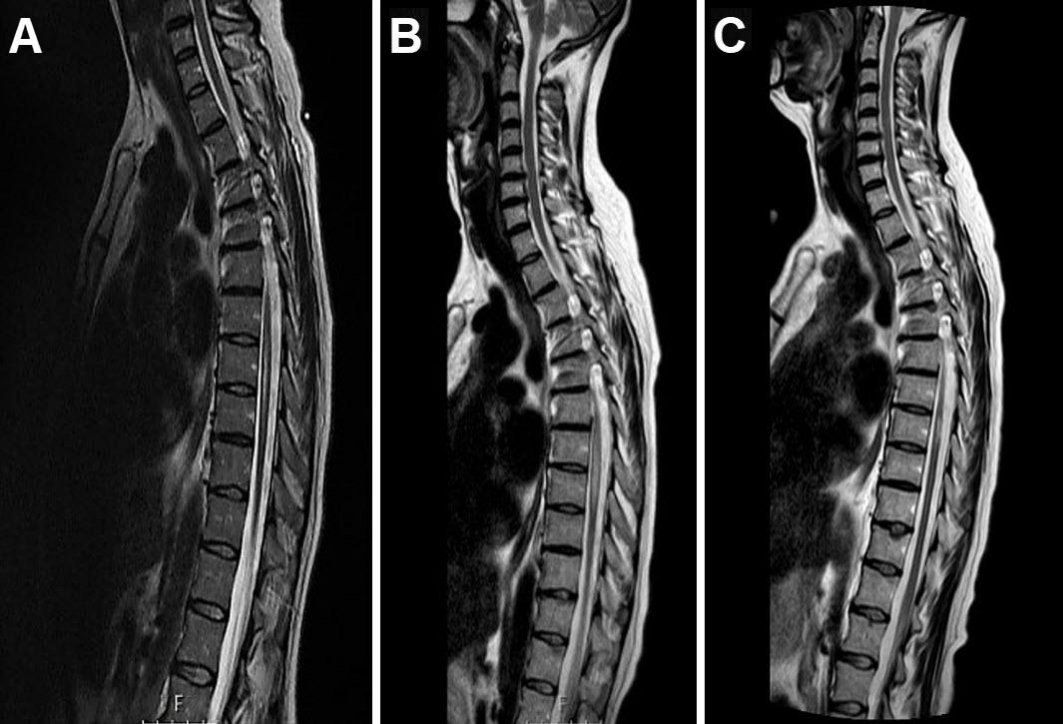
During Prednisone Taper, the Patient Presented with Sensory Loss in Both Legs. Neurological examination demonstrated a D7 sensory level. Spinal MRI revealed an inflammatory longitudinal myelitis lesion extending from D7 to D11, here shown on sagittal T2 of the dorsal spine (A). The patient was treated with induction therapy followed by monthly pulsed i.v. cyclophosphamide infusions. After the 5th infusion, the patient developed para-paresis, urinary incontinence, and sensory level above her legs. A spinal MRI demonstrated a new longitudinal lesion extending D6–D9 (B). Neuro-ophthalmologic studies were negative. Anti-aquaporin 4 antibodies (AQP4) were negative at that time. Induction therapy was re-instituted; maintenance with azathioprine and high-dose IVIg was initiated. The patient’s condition stabilized, and she remained with minimal left-hand paresis and mild spinal ataxia and sensory loss, with improvement visible on repeat MRI (C).
